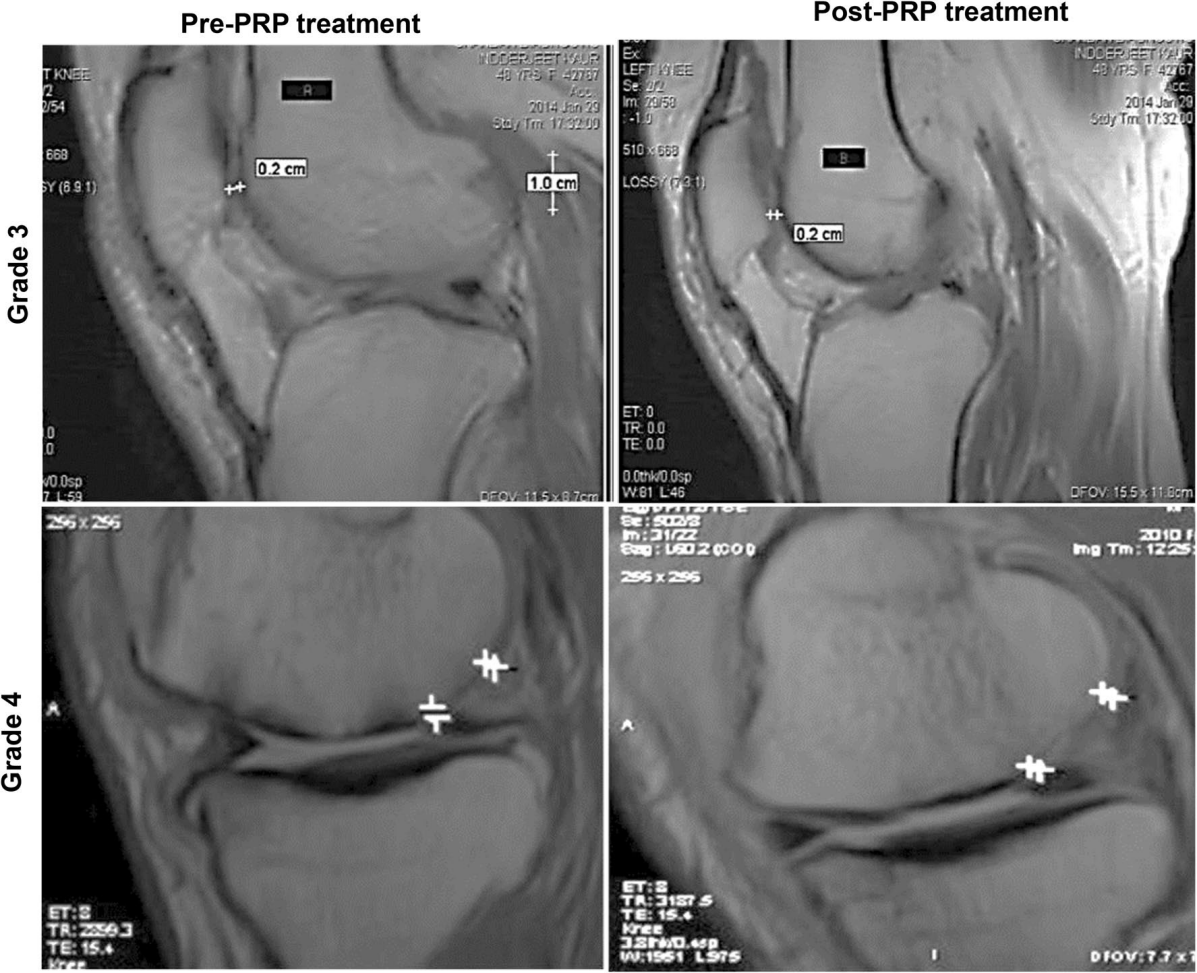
Evaluation of articular cartilage thickness using MRI after one year of PRP treatment in patient with grade 2 and grade 3 OA knee.
